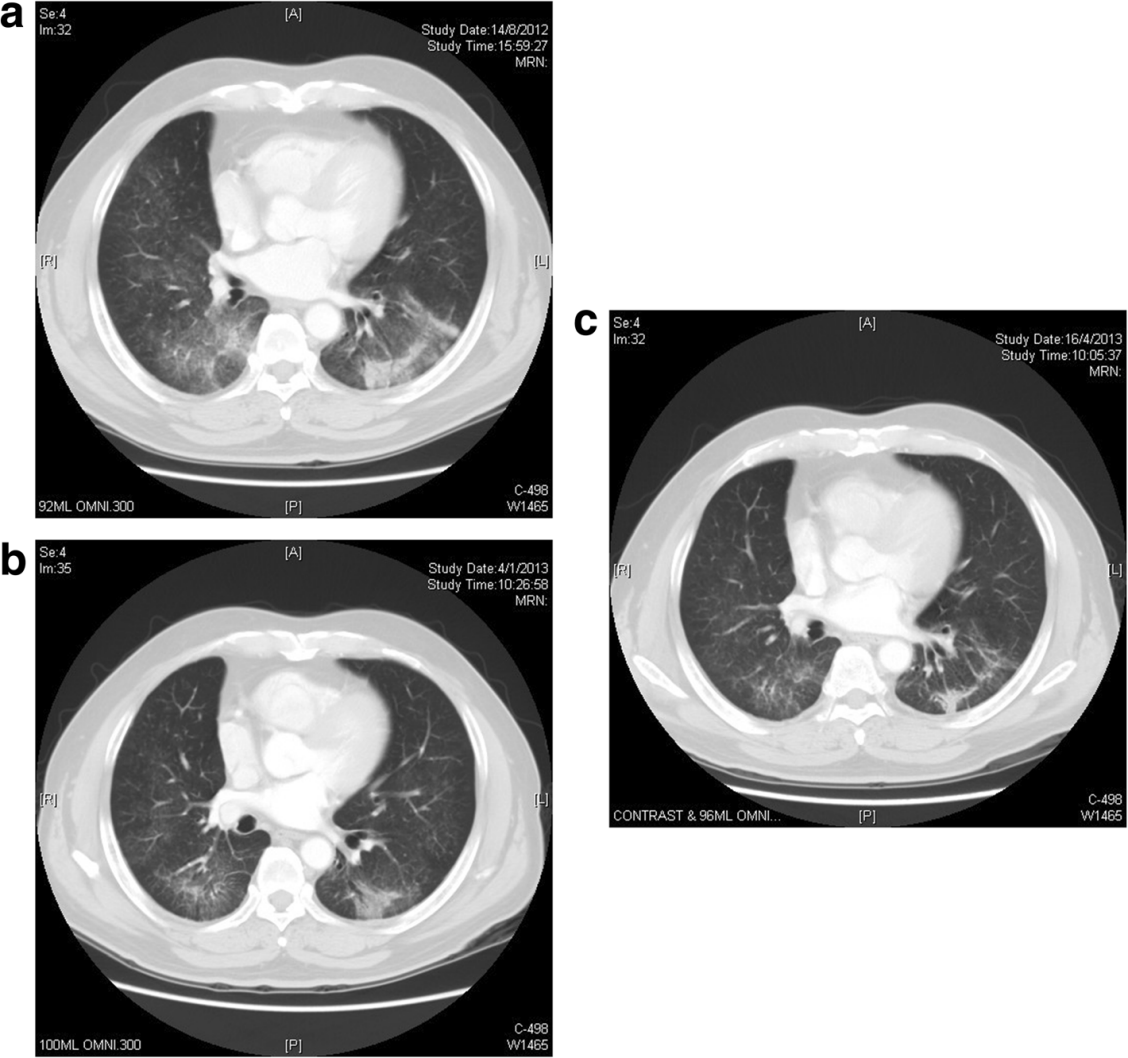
Computed tomography images of one of our study patients with metastatic bronchoalveolar carcinoma which harbored exon 19 deletion treated with afatinib as 2^nd^ TKI therapy after failure to gefitinib and chemotherapy. a. Baseline images showing diffuse ground glass opacities representing tumor infiltrates in lower lobes of both lungs. b. CT images at 3 months after afatinib showing significant reduction of tumor infiltrates. c. CT images at 6 months after afatinib showing further response and tumor shrinkage to afatinib
